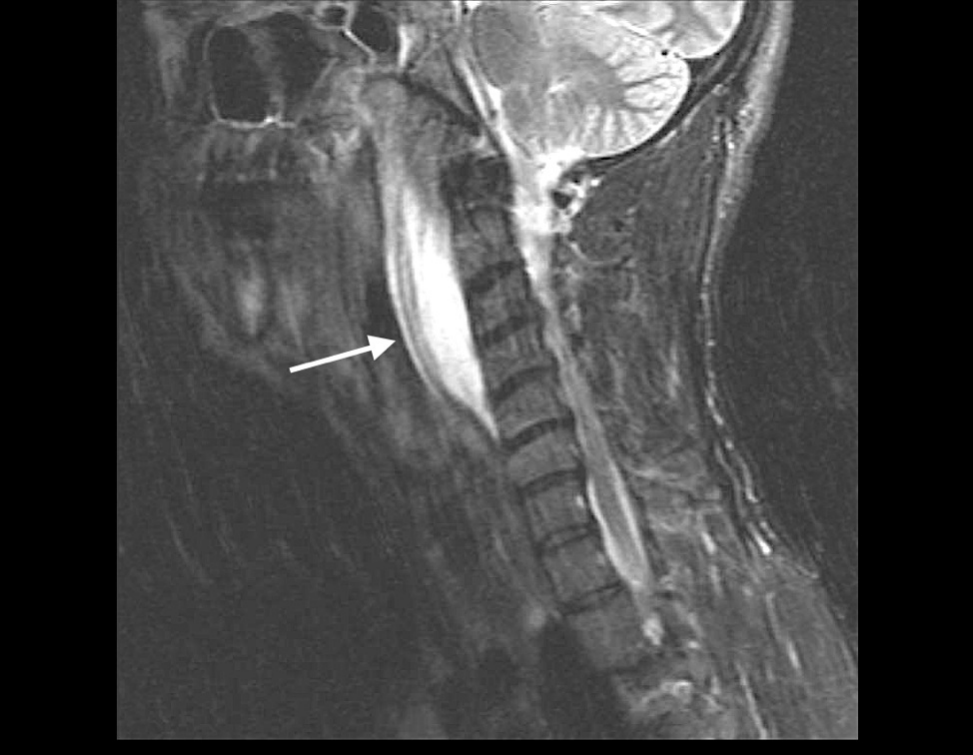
Magnetic resonance imaging (MRI) of soft tissues of the neck showing a retropharyngeal abscess (white arrow)
